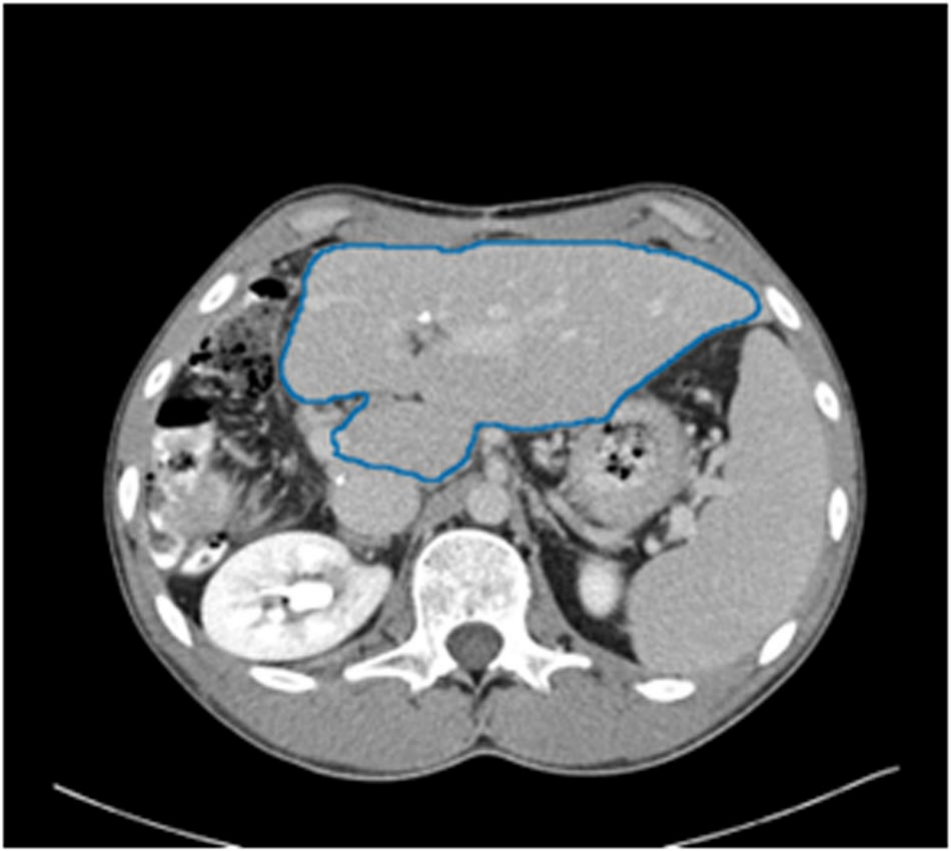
Automatic segmentation of the liver from CT scan of patient TCGA-DD-A1EH.
